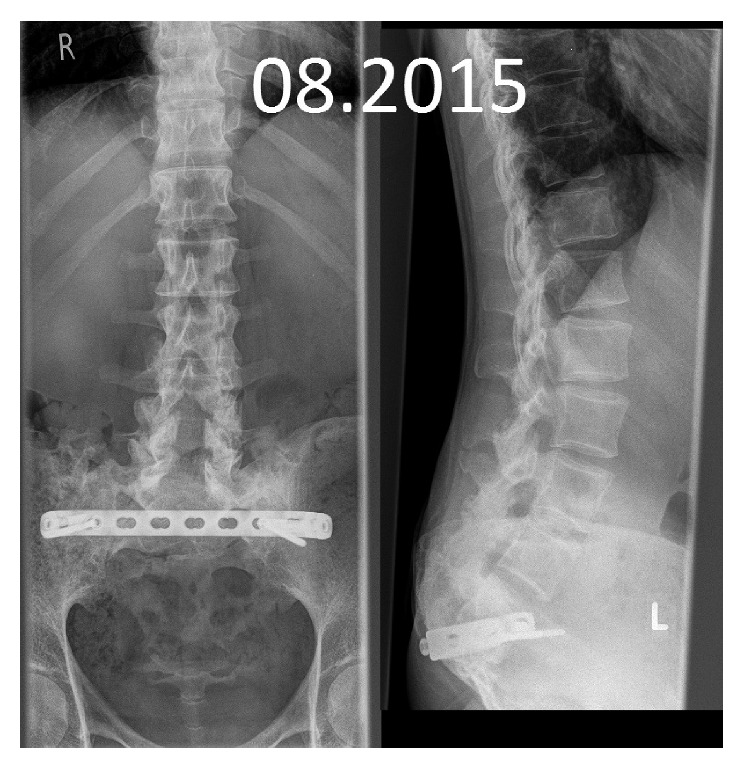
X-ray control in the lower spine.
